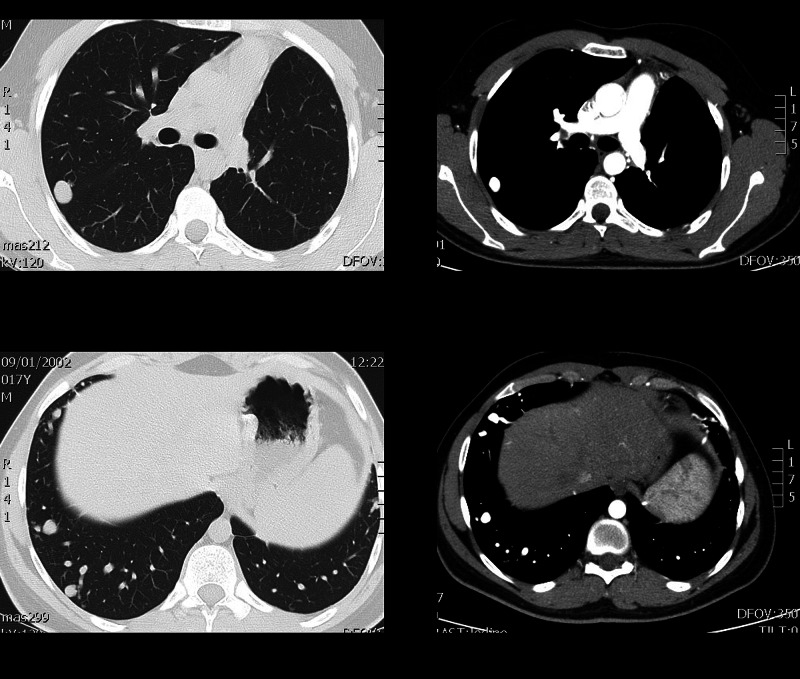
Axial CT images in the lung and soft tissue window show a large PAVM with a proximal tail-like extension and many smaller arteriovenous malformations. PAVM, pulmonary arteriovenous malformation
